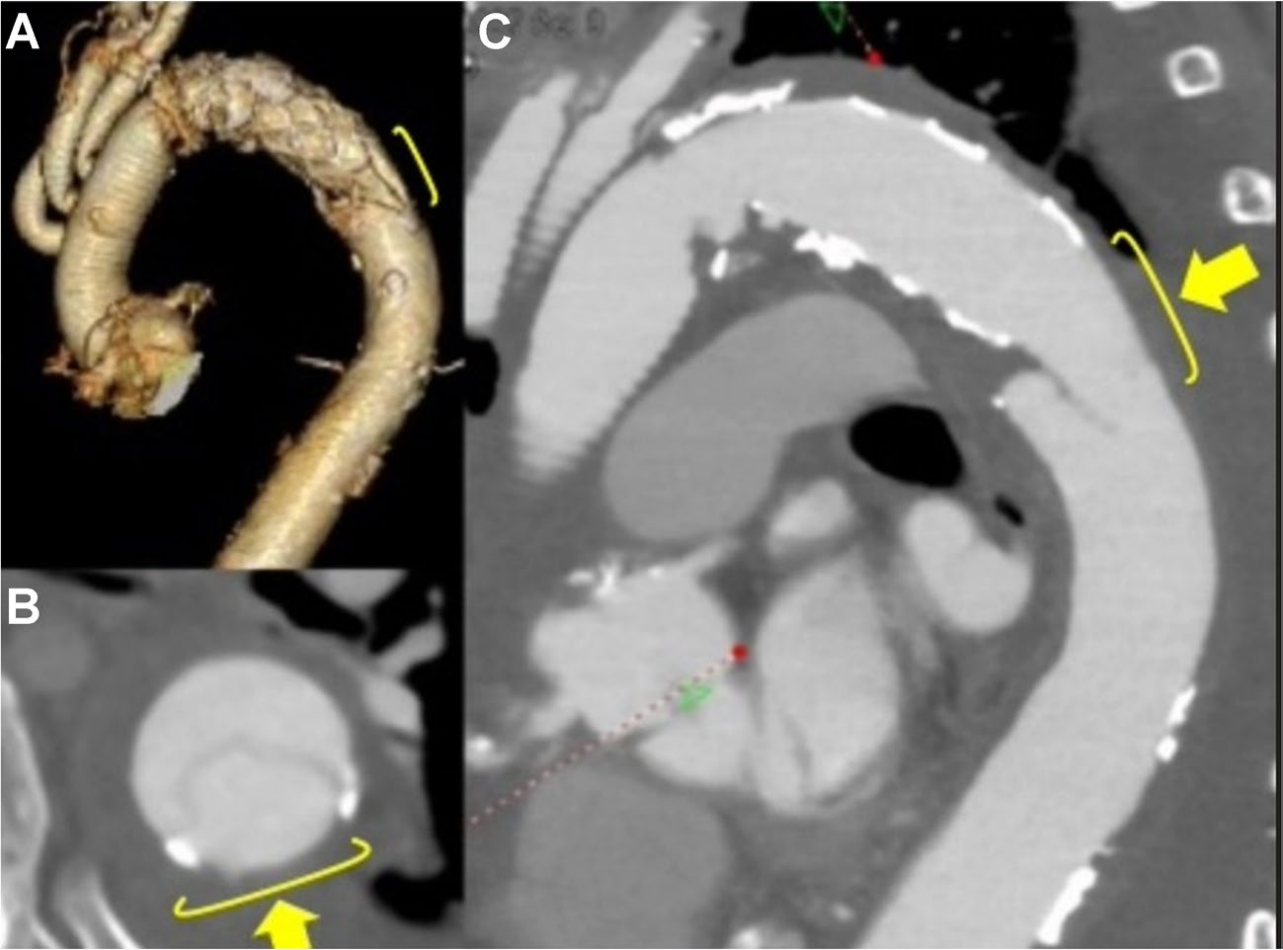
Representative presentation of postoperative aortic computed tomography angiogram featuring J Graft Frozenix Partial ET. A Three-dimensional reconstruction, B axial view, and C sagittal view. The yellow arrows and lines denote the distal unstented segment, which exhibits a slightly tapered configuration and demonstrates adequate contact with the major curvature side of the aorta
